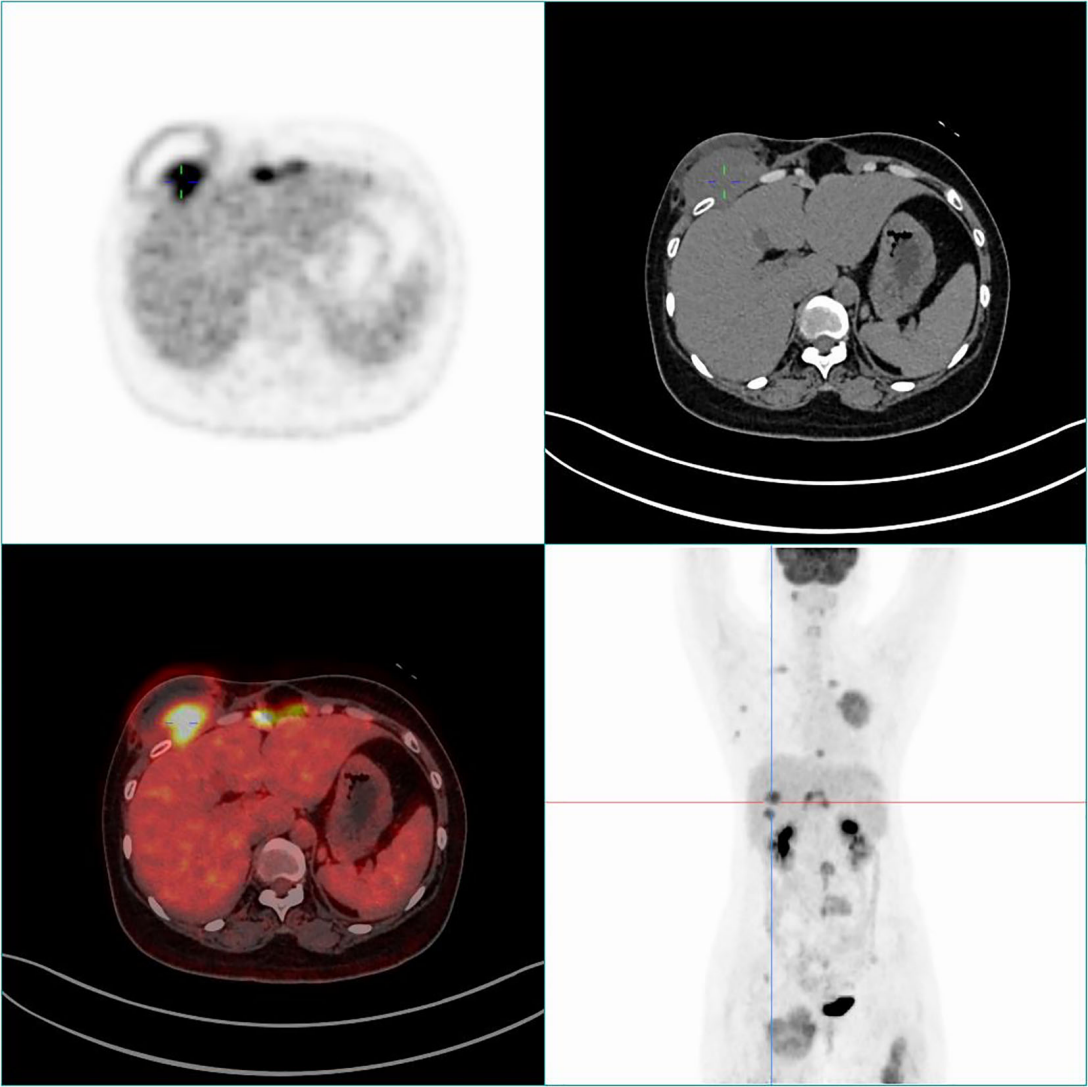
PET/CT scan of subcutaneous soft tissue metastasis in the right anterior chest of a 40 year old female patient. The whole-body maximum intensity projection (MIP) and fusion PET/CT and CT axial images showed an FDG positive nodule in the right anterior chest (SUVmax=8.8). Pathological indication: malignant schwannoma.
